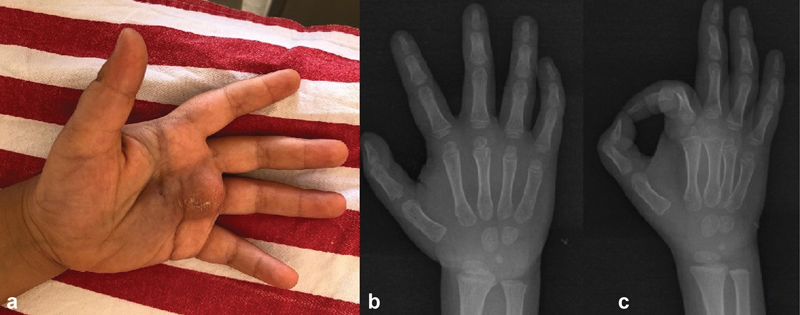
a) immobile, pasty soft tissue mass in the palm of the left hand. b,c) No pathological finding was detected in direct radiographs.
